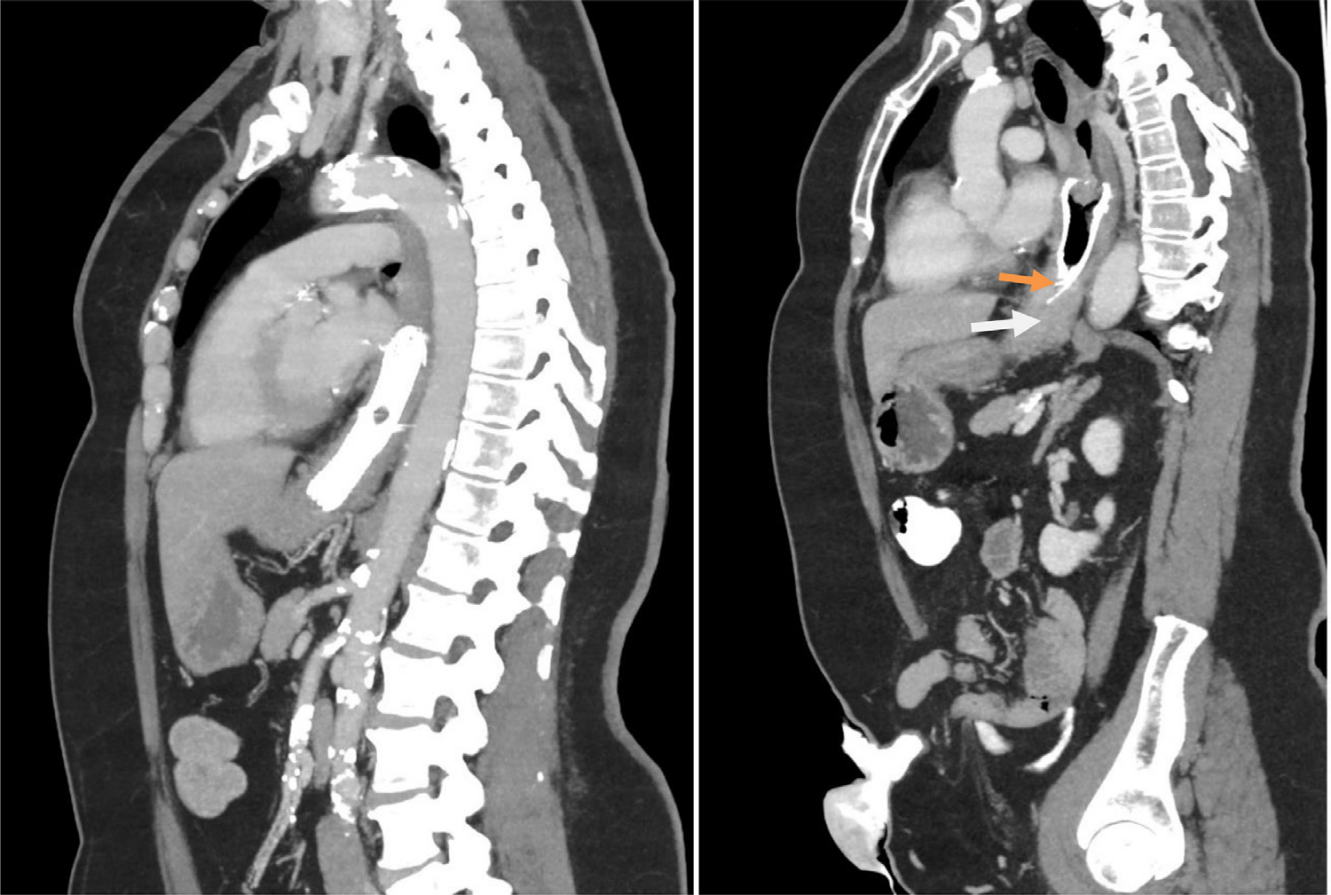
CT on the left performed 2 months prior to contrast swallow demonstrating intact stent and CT on the right immediately following contrast swallow demonstrating stent fracture (orange arrow) and tumor ingrowth (white arrow).
